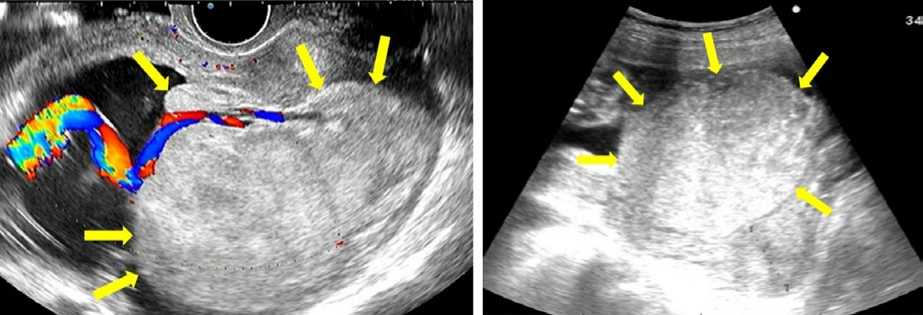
Ultrasonographic image shows (arrows) a spherically enlarged placenta; color Doppler sonography shows the umbilical cord
